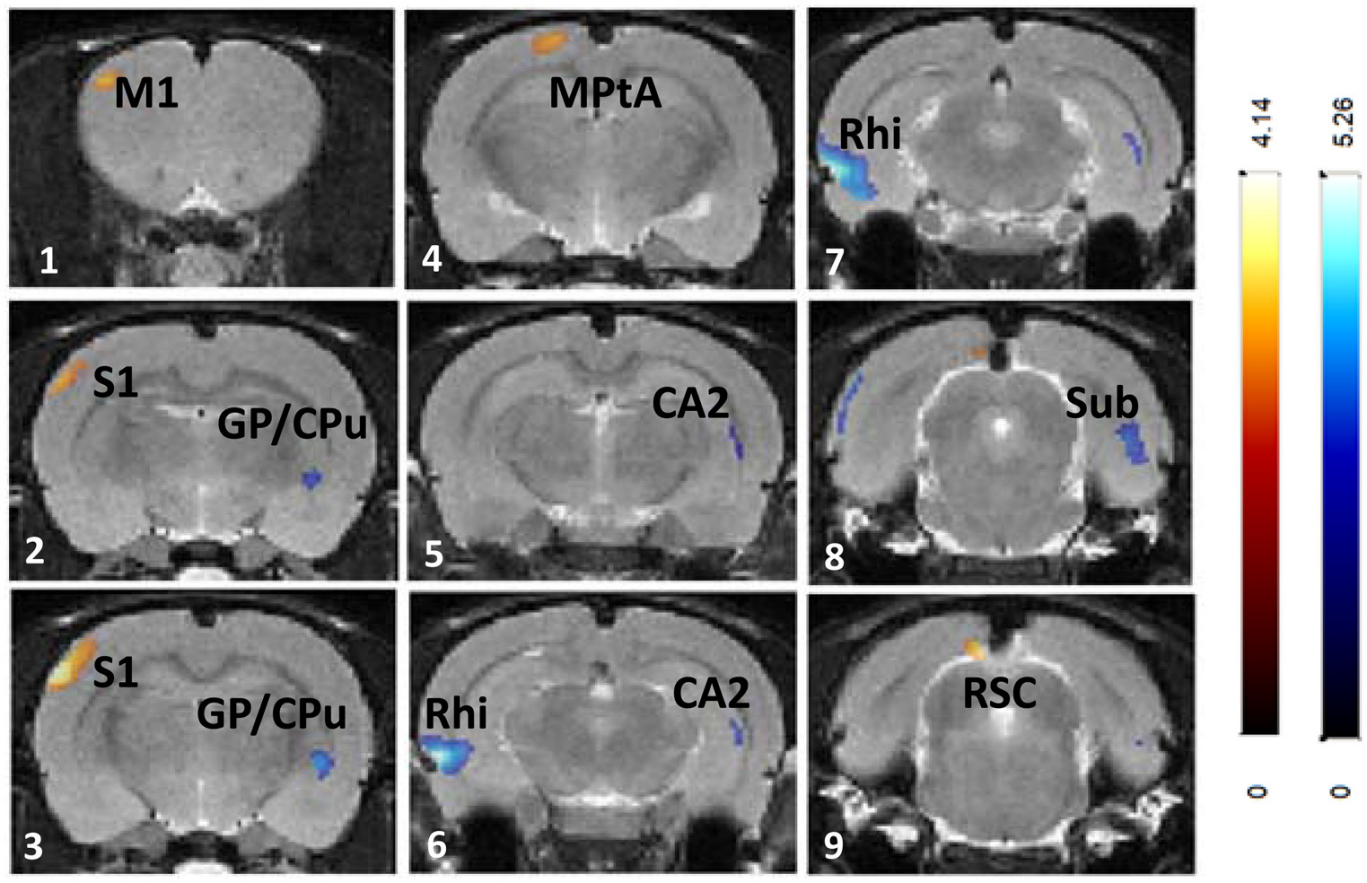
Effects of chronic HD MA (8mg/kg) treatment on regional brain glucose metabolism. Changes in regional brain glucose metabolism are superimposed on the Schweinhardt MRI template, in Paxinos space. Red: brain regions with increased glucose metabolism after 8mg/kg MA treatment compared with vehicle treatment; Blue: brain regions with decreased glucose metabolism after MA 8mg/kg treatment compared with vehicle treatment. M1: primary motor cortex; S1: primary somatosensory cortex; CPu: caudate-putamen (striatum); GP: globus pallidus; PtA: parietal association area; CA1: cornu ammonis 2 (hippocampus); Rhi: rhinal cortices; Sub: subiculum; RCS: retrosplenial cortex.
